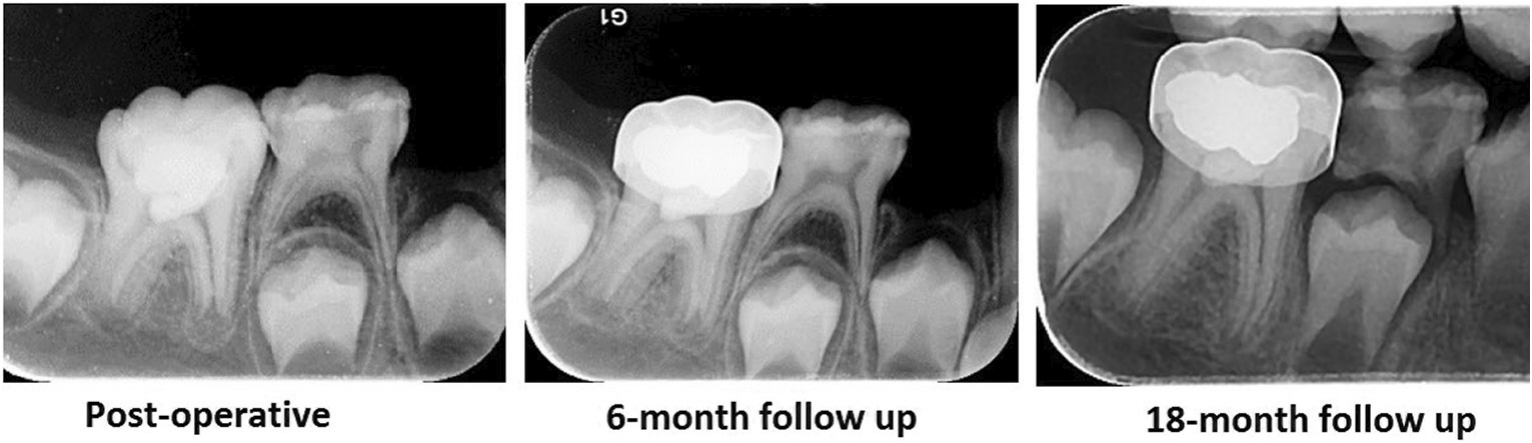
Post‐operative and follow‐up radiographs of tooth #30 after vital pulp therapy.
